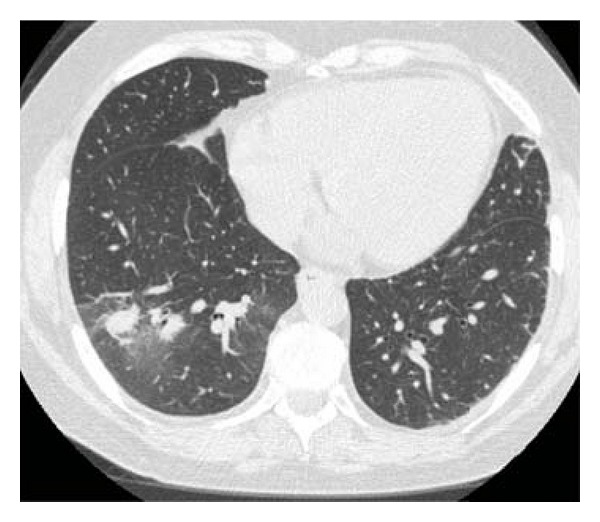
Chest CT scan without intravenous contrast on initial admission showing patchy infiltrates and a “halo sign” surrounding a right lower lobe lesion.
